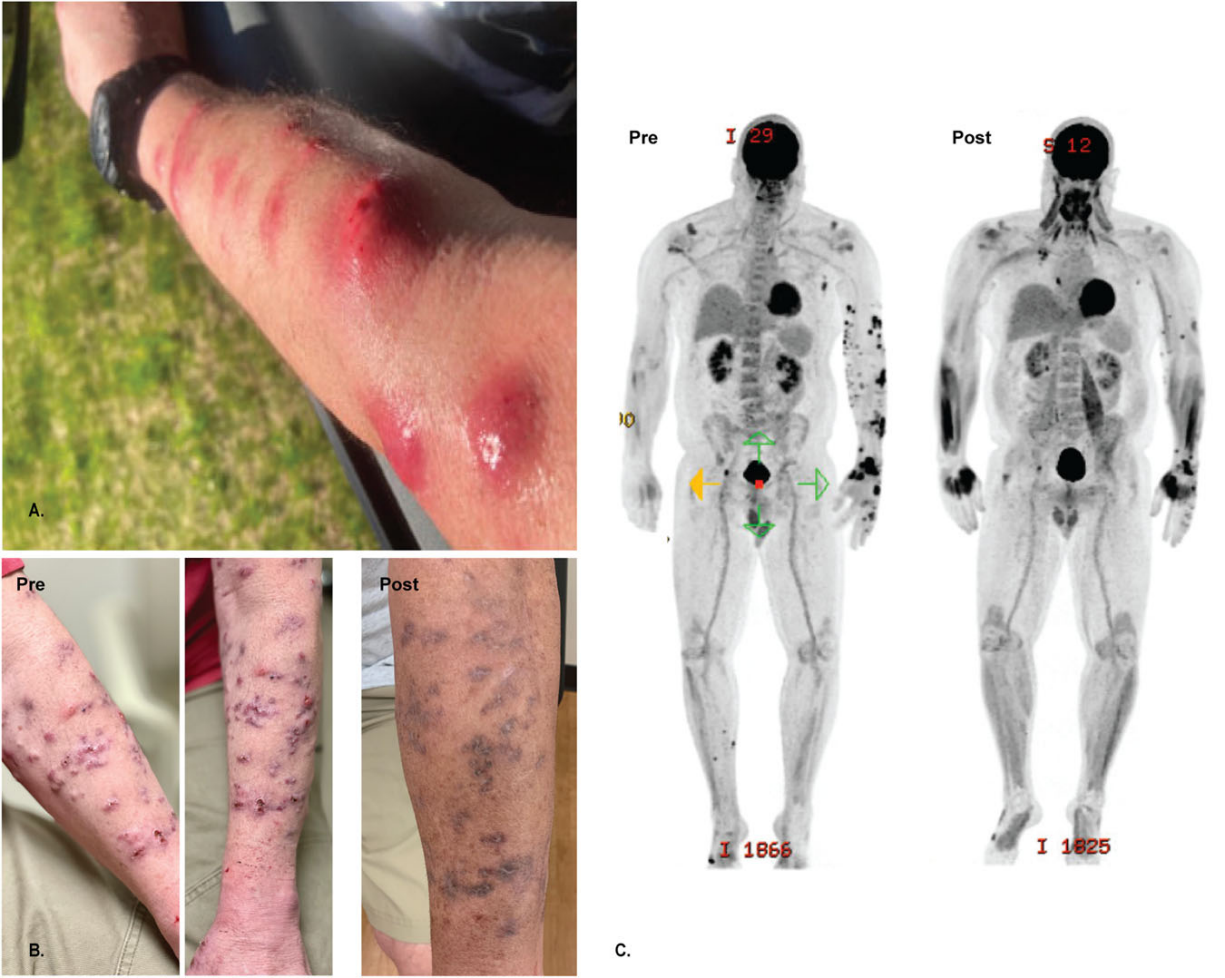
Clinical course of *M. chelonae* infection. A Left upper extremity with multiple large erythematous, fluctuant to nodular lesions ultimately diagnosed as disseminated cutaneous *Mycobacterium chelonae* infection. B Images of the left upper extremity lesions prior to (Dec 2020) and following (August 2021) addition of bacteriophage therapy. C PET/CT prior to (March 2021) and following (August 2021) addition of bacteriophage therapy.
